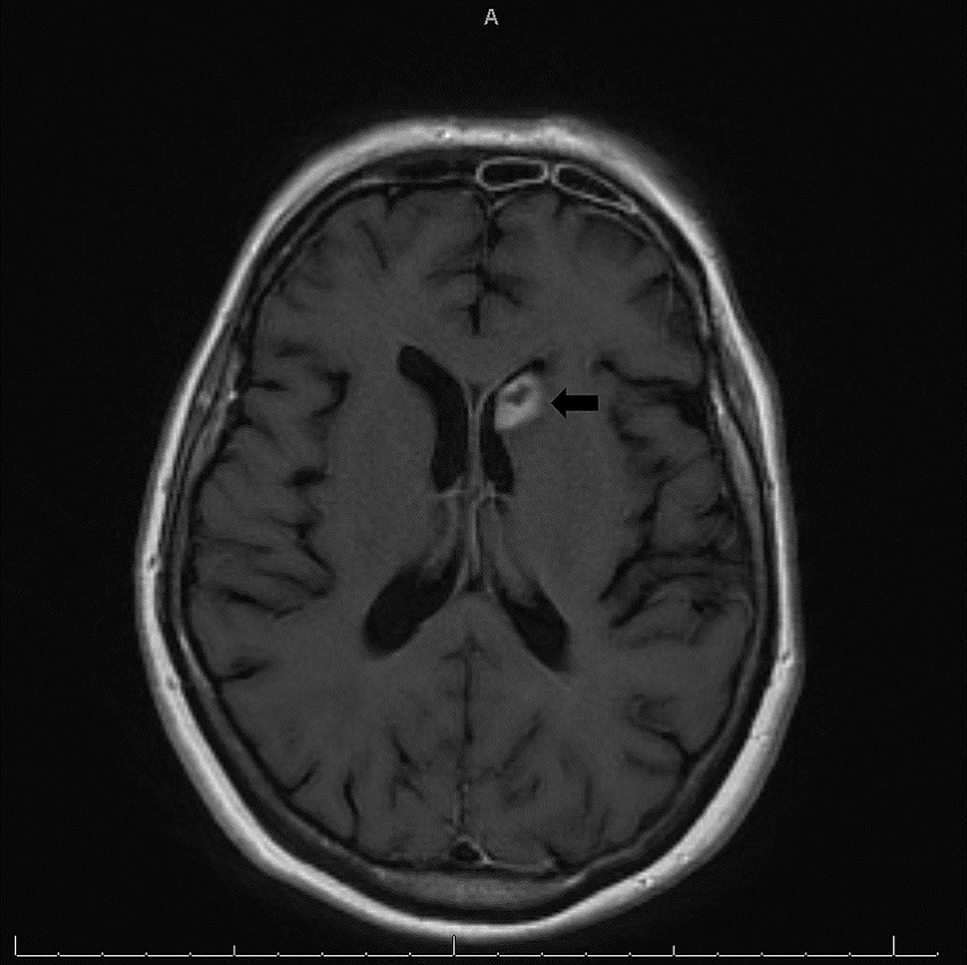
T1-weighted magnetic resonance image (MRI) with contrast medium showing a 1.6 cm rim enhancing lesion in the left caudate with surrounding edema and probable hemorrhagic components
